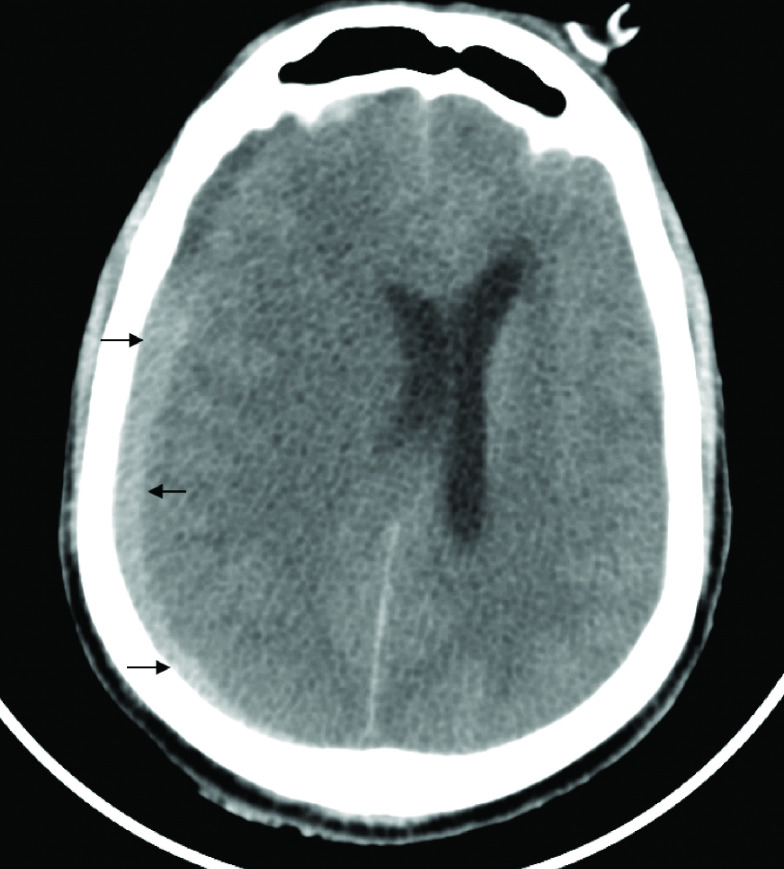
Subdural haemorrhage in case 2. Computed tomography head of patient B showing biconcave hyperdensity in the right parieto-occipital location with associated midline shift suggestive of subdural hemorrhage.
